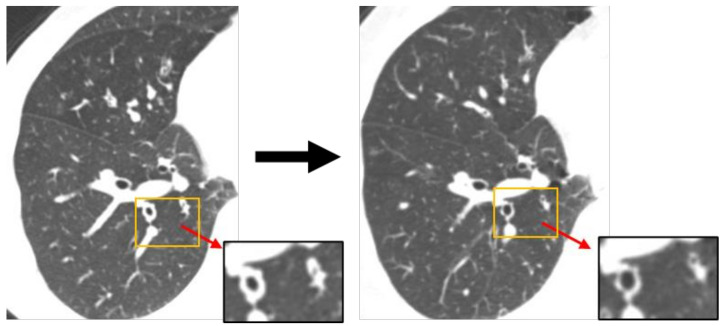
Chest CT before and one year after the start of dupilumab.
